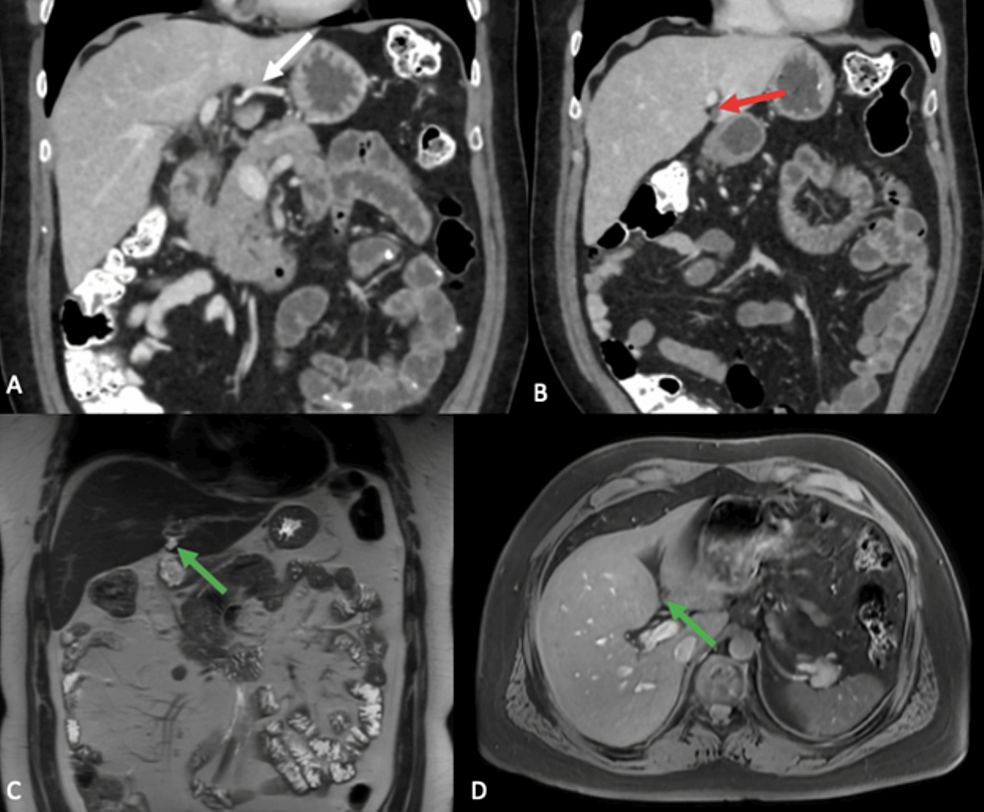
Contrast-enhanced cross-sectional imaging Portal phase contrast-enhanced abdominal CT (A, B) showing an aberrant course of the left gastric vein (white arrow) draining into the posterior margin of the hepatic segment III, consistent with an ALGV. A focal hypodense perfusion defect traversed by blood vessels in its posterior aspect, compatible with an HPS is also found in hepatic segment III (red arrow). A contrast-enhanced MRI (C, D) showed the same perfusion defect (green arrows) compatible with an HPS, as hyperintense on T2 and hypointense on T1. ALGV: aberrant left gastric vein; HPS: hepatic pseudolesion
